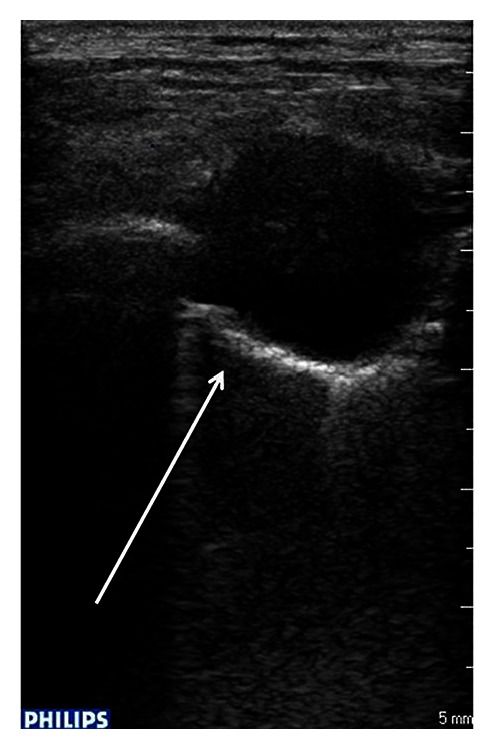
Rounded lesion involving the pleura. Chest ultrasonography showing a rounded lesion involving the pleura with rear-wall reinforcement and a localized alveolar-interstitial syndrome (arrow).
